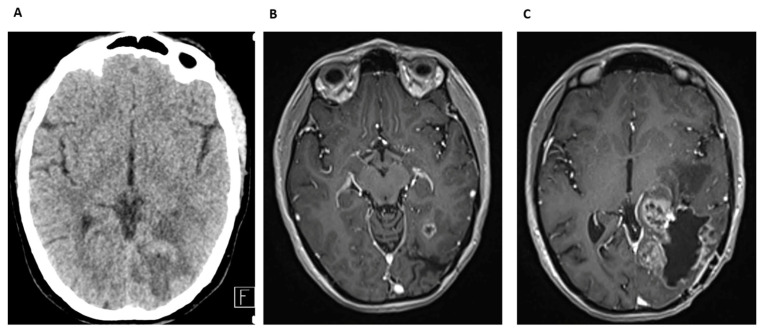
Cranial CT and MRI scan of patient 3. (A) Preoperative native CT axial image; (B) postoperative routine T1 contrast-weighted axial image; (C) postoperative routine T1 contrast-weighted axial image with tumor progression.
